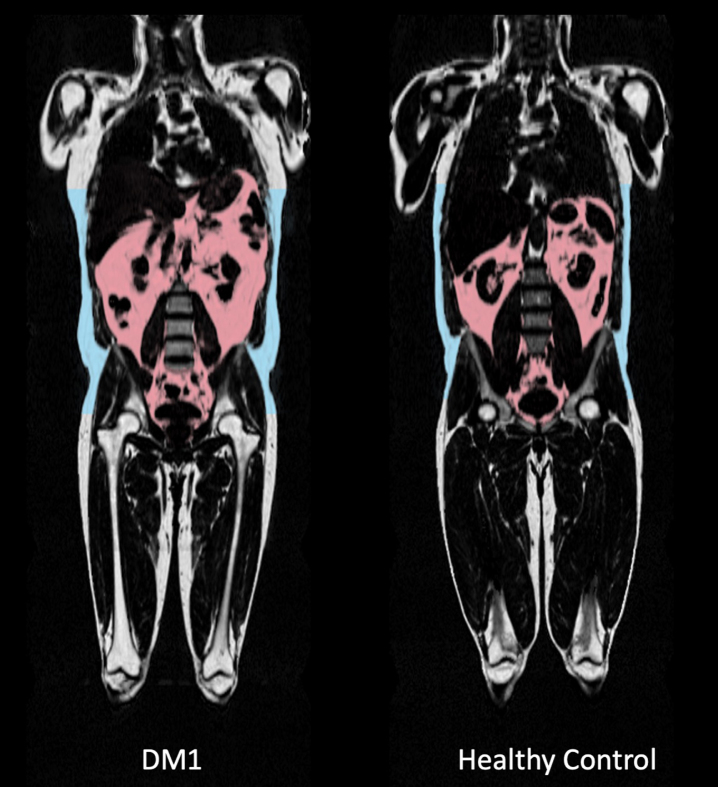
Comparison of full body MRI images between a myotonic dystrophy type 1 (DM1) patient and a healthy age-, sex- and BMI-matched control. Visceral adipose tissue (VAT) deposition is highlighted in pink. VAT volume was 5,59L in the displayed DM1 patient versus 4,28 L in the healthy matched control. Subcutaneous fat deposition is highlighted in blue, as part of the total adipose tissue volume. Also note the difference in shoulder and leg musculature: thigh lean muscle volume was 11,1 L in the DM1 patient versus 15,1 L in the healthy control, as part of total lean tissue volume.
